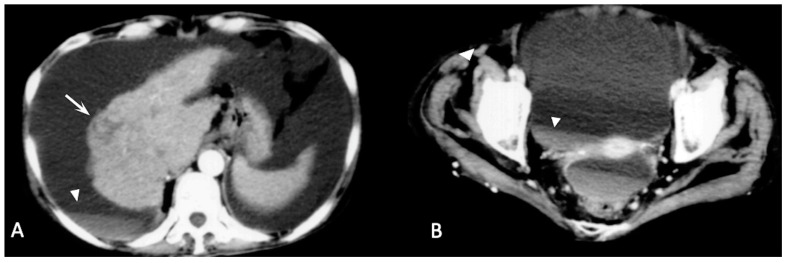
Arterial-phase enhanced CT scan. Protruding subcapsular HCC of the VIII segment with intra-tumoral necrosis and a slight enucleation sign (arrow). Peri-hepatic and peri-splenic effusions are also visible. Peri-hepatic fluid is contaminated by blood in the dependent site (hematocrit sign, arrowheads (A,B)).
